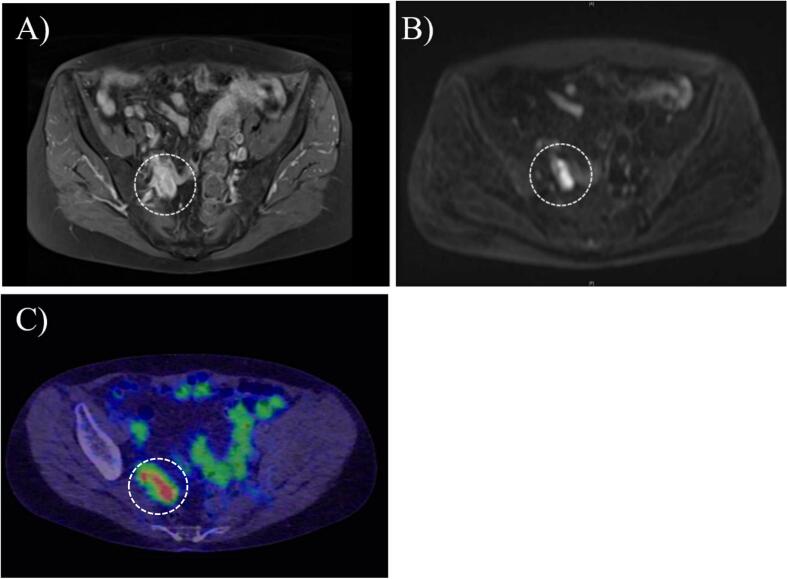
(A) Pelvic magnetic resonance imaging (MRI). Gadolinium-enhanced T1-weighted image. A contrast-enhancing tubular mass is observed in the right adnexal region. The lesion is outlined. (B) MRI. Diffusion-weighted image (DWI). The tubular mass exhibits high signal intensity on DWI. The lesion is outlined. (C) FDG-PET/CT showing increased FDG uptake (SUVmax 9.81) in the right adnexa.
